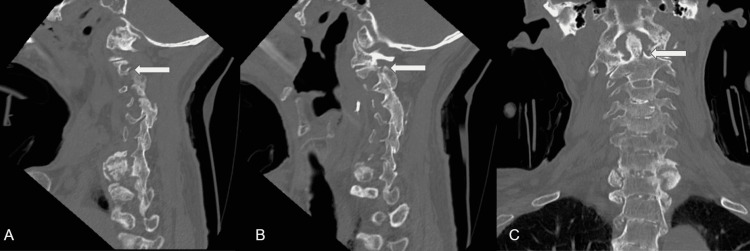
Left (A) and right (B) preoperative sagittal view CT scan of the facet fracture. Preoperative coronal view CT scan of the C2 dens/body fracture (C) CT: computed tomography
